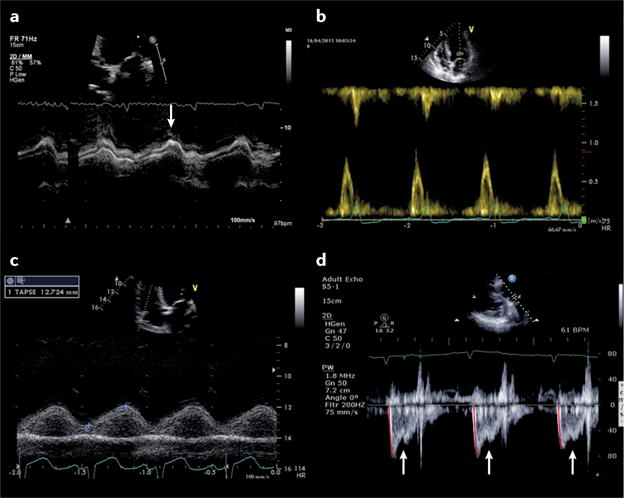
The haemodynamic effects of thrombosis (coronary and pulmonary) as demonstrated by echocardiography a | Early features of myocardial ischaemia can be demonstrated by the presence of prolonged long-axis shortening, measured by M-mode echocardiography across the base of the left ventricle (post-ejection shortening; arrow). b | Prolonged left ventricular wall tension suppresses early transmitral filling, resulting in an isolated late-diastolic transmitral A wave. c | Increased right ventricular afterload leads to a reduction in right ventricular systolic function, as demonstrated by tricuspid annular plane systolic excursion on M-mode echocardiography across the tricuspid annulus. d | A substantial increase in pulmonary vascular resistance might be associated with a midsystolic notch (arrows) on pulmonary valve pulsed-wave Doppler ejection wave and a short pulmonary valve acceleration time (78 ms; red lines).
